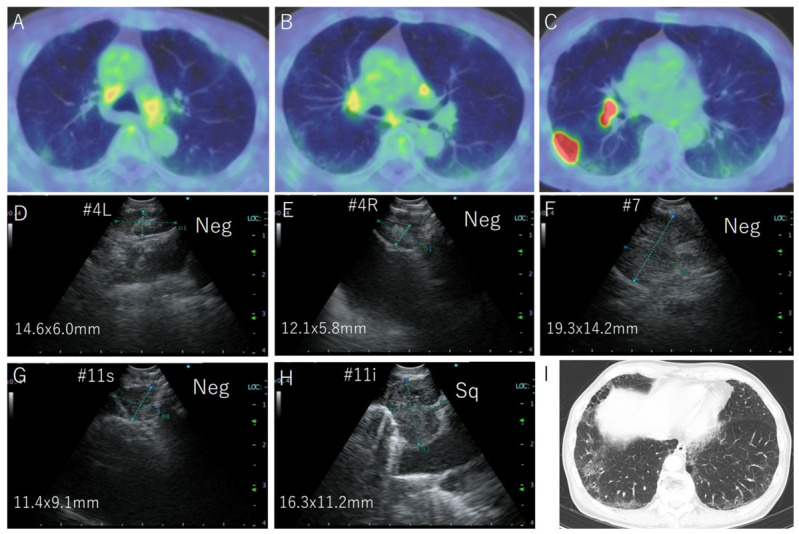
False-positive N3 findings on PET-CT in a patient with right lower lobe lung cancer. The primary tumor located in the right lower lobe was diagnosed as squamous cell carcinoma. The patient had comorbid interstitial pneumonia (I). FDG-PET/CT showed multiple FDG-avid mediastinal lymph nodes, suggesting N3 disease (A–C). EBUS-TBNA was subsequently performed, and samples obtained from stations 4 L, 4R, 7, and 11s revealed epithelioid cells suggestive of a sarcoid-like reaction (D–G). Only station 11i was positive for metastatic squamous cell carcinoma (H), resulting in a final diagnosis of N1 disease, and the patient underwent perioperative chemoimmunotherapy. Neg: negative; Sq: squamous cell carcinoma.
